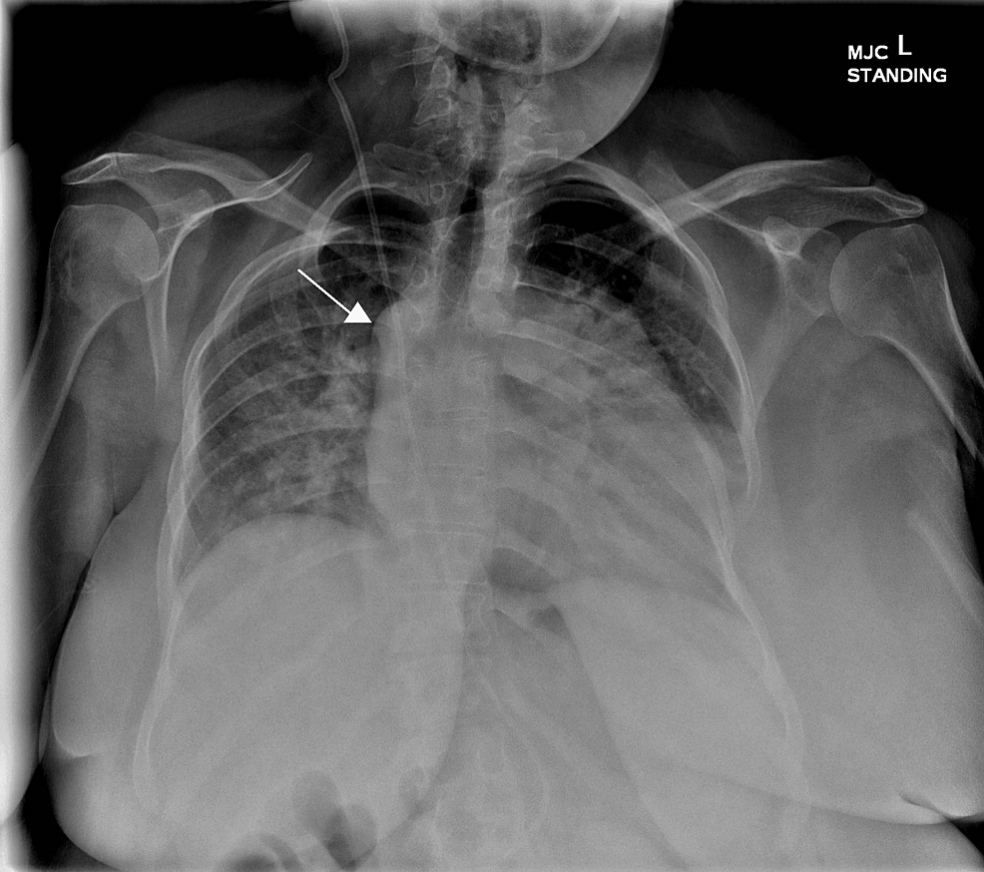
Chest x-ray on admission with pulmonary congestion In addition to the increased interstitial markings, note the intact ventriculoperitoneal shunt tubing. White arrow points to enhancement of the arch of the azygous vein where it joins the superior vena cava. Azygos continuation of the inferior vena cava has long been associated with congenital heart disease and asplenia or polysplenia (this patient has both CHD and polysplenia), but with the increased use of cross-sectional imaging, it is increasingly being recognized in asymptomatic and otherwise anatomically typical individuals.
